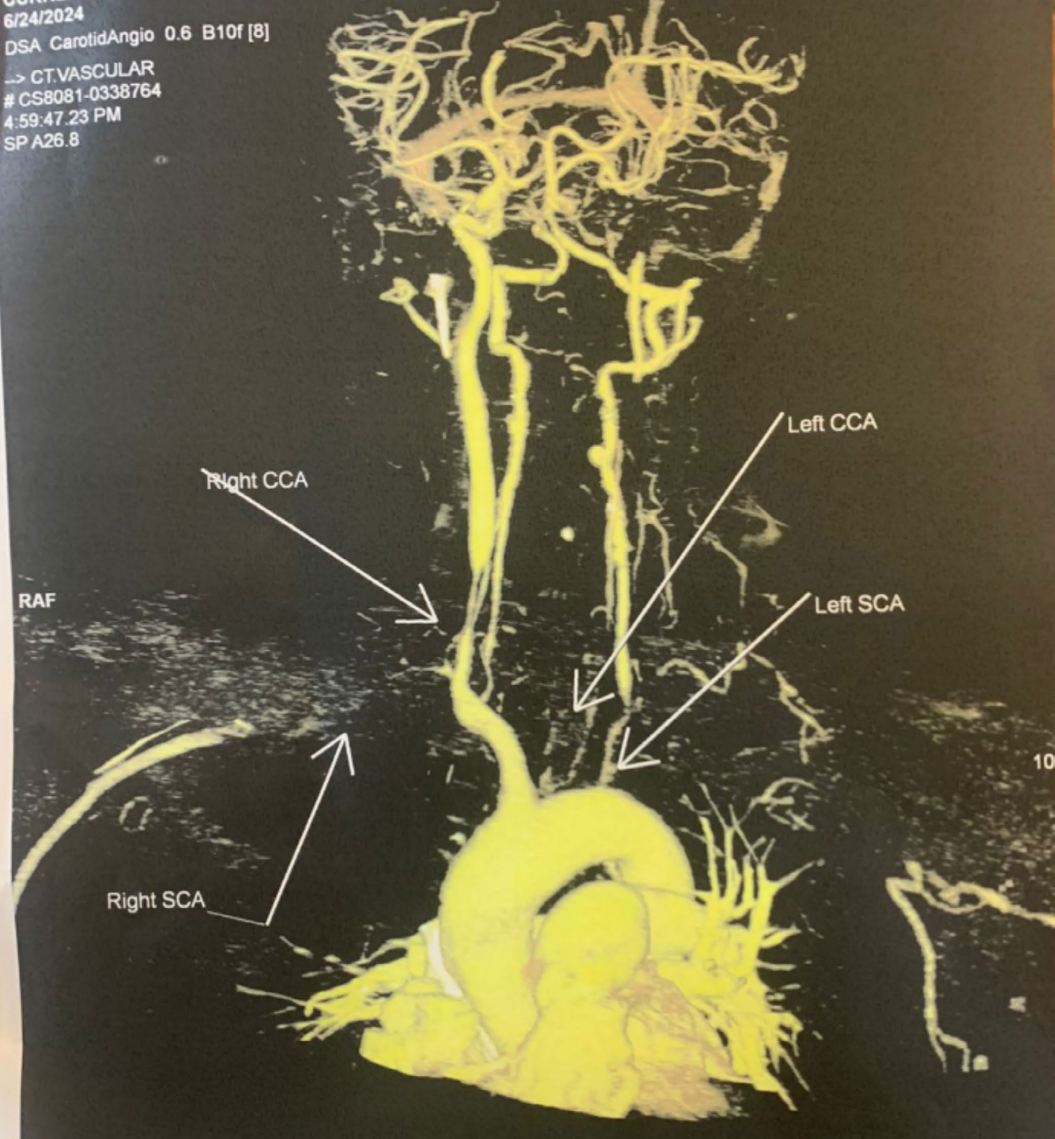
Carotid CT angiogram demonstrating circumferential thickening of all branches of arch of aorta.
